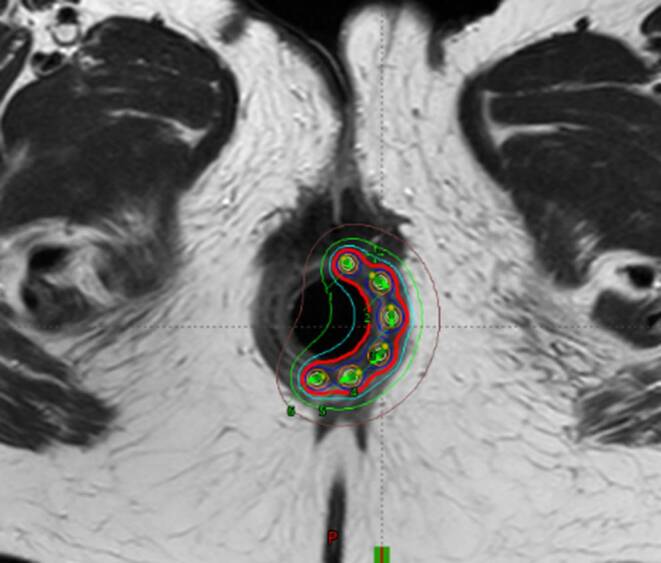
Dose distribution analysis on the post implant MRI scan
